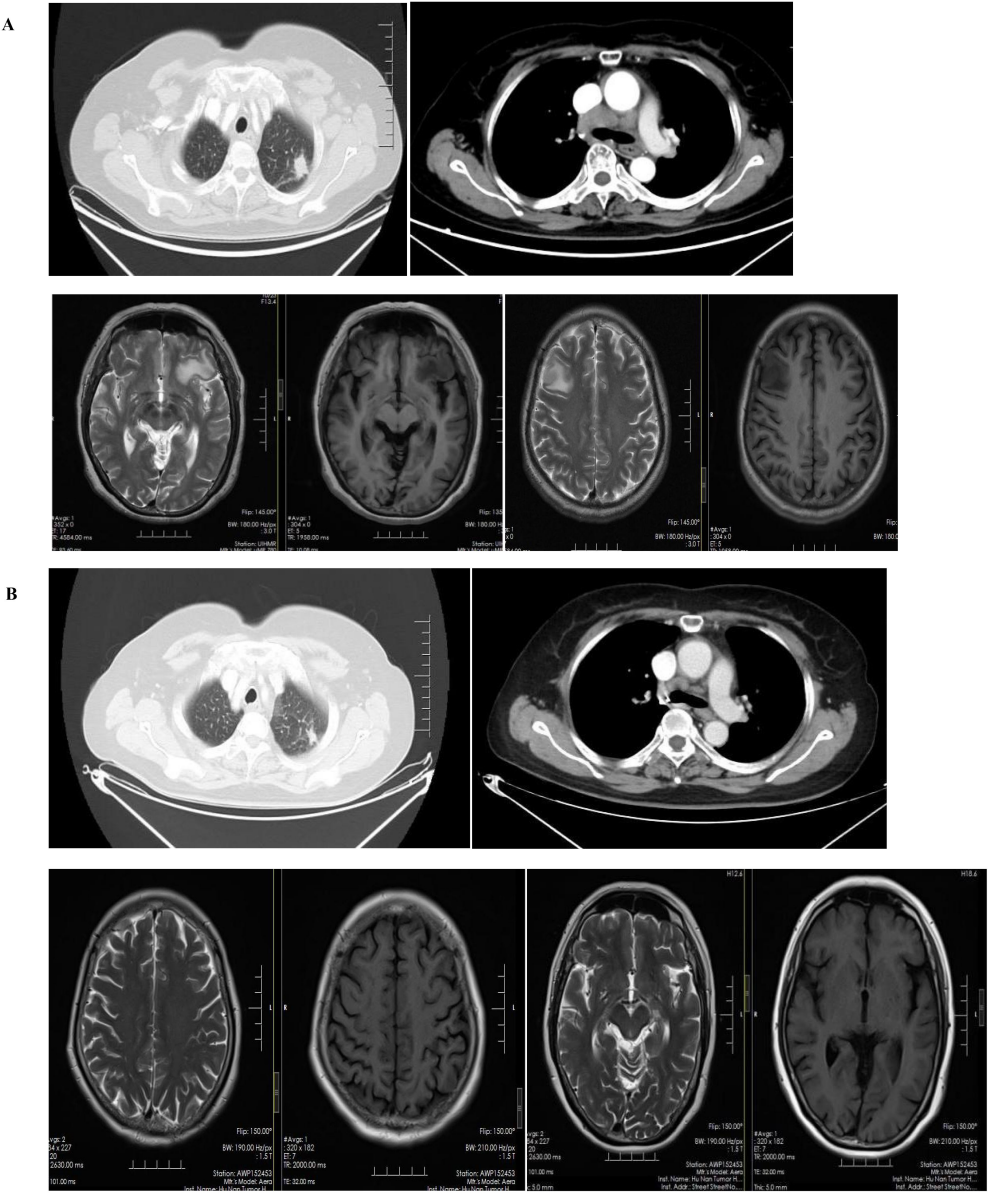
Radiographic alterations in the patient. (A): Multiple brain metastases, pulmonary lesions and mediastinal lymphadenopathy before treatment. (B): comparison of lung and intracranial lesions after treatment.
